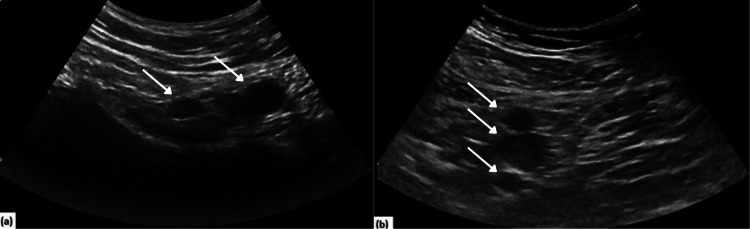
Ultrasound of the inguinal regions shows scattered hypoechoic macrolobulated lymph nodes. These lymph nodes have lost their expected fatty hilum. The largest lymph node on the right (a) measures 2.3 x 2.2 x 1.7 cm, and the one on the left (b) measures 2.4 x 1.8 x 1.1 cm.
